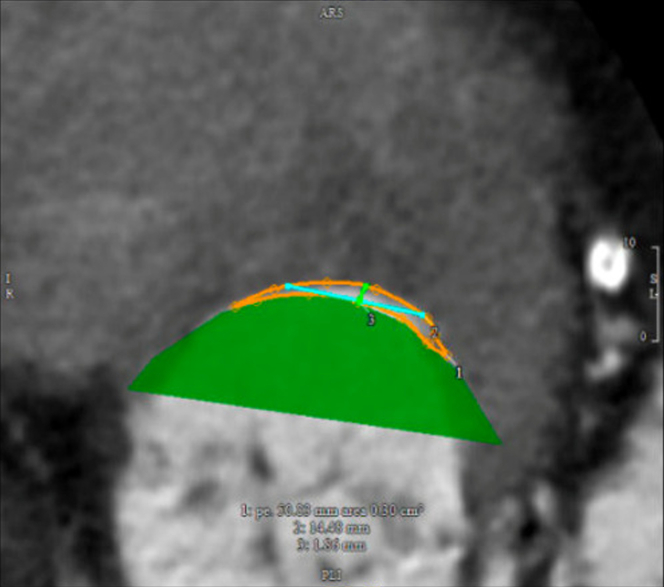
Cardiac CT showing prohibitive predicted neo-LVOT area of 0.30 cm^2^ for TMVR with ViMAC. Abbreviations: CT, computed tomography; LVOT, left ventricular outflow tract; TMVR, transcatheter mitral valve replacement; ViMAC, valve-in-mitral annular calcification.
